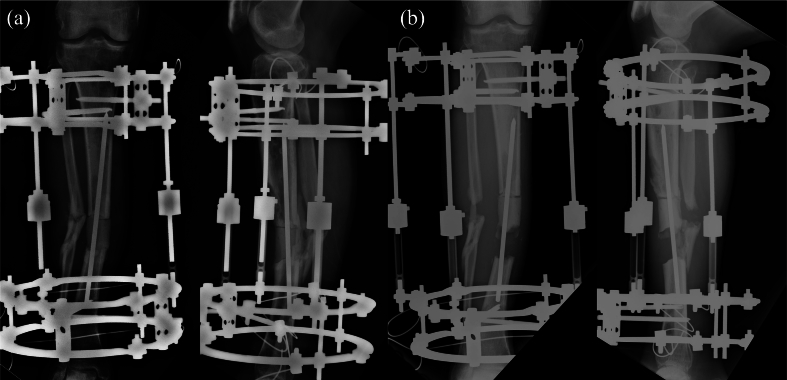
Radiographic images during (a) application of the Ilizarov external fixator following angular deformity realignment and (b) soft tissue lengthening at a rate of 3 mm/day.
